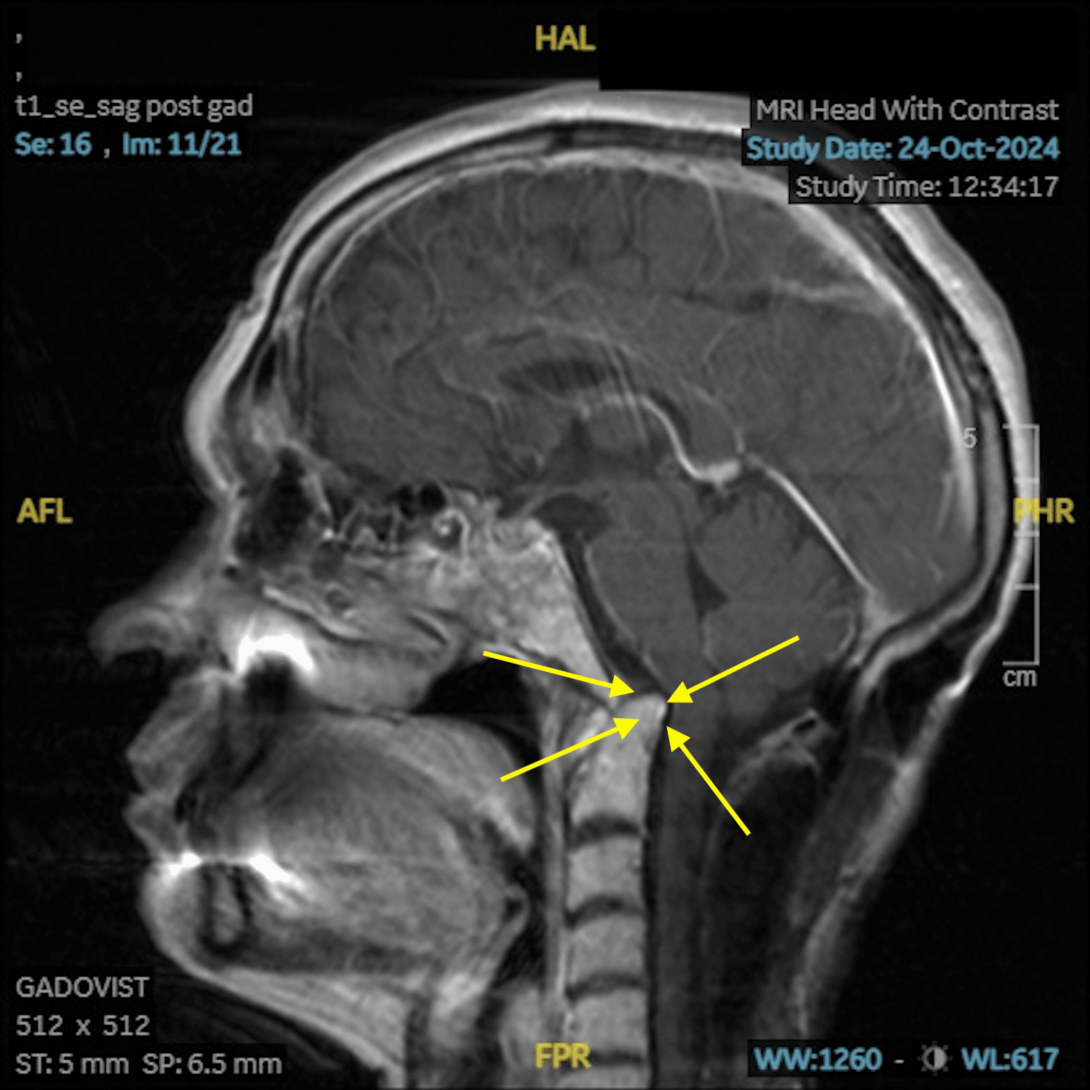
Sagittal T1-weighted post-contrast MRI Sagittal T1-weighted post-contrast MRI of the brain and upper cervical spine showing basilar invagination, with superior displacement of the odontoid process into the foramen magnum, resulting in cervicomedullary junction narrowing.
